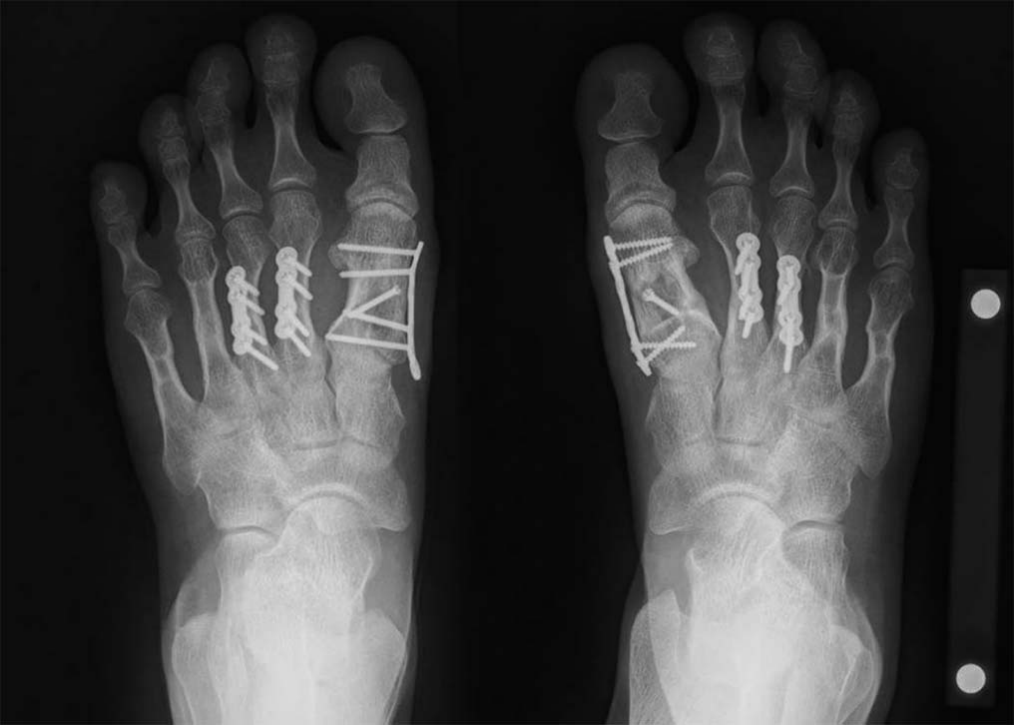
Radiograph made 1 year after 1-stage step-cut lengthening of the first metatarsal (which resulted in an increase of 12 mm [or 34% of the original length] in the right foot and 11 mm [31% of the original length] in the left foot), second and third metatarsal shortening (which resulted in 5 mm and 5 mm of shortening, respectively, in the right foot and 6 mm and 4 mm of shortening, respectively, in the left foot), and 1-stage lengthening of the fourth and fifth metatarsals with use of interpositional grafts from the second and third metatarsals that were fixed with intramedullary Kirschner wires.
